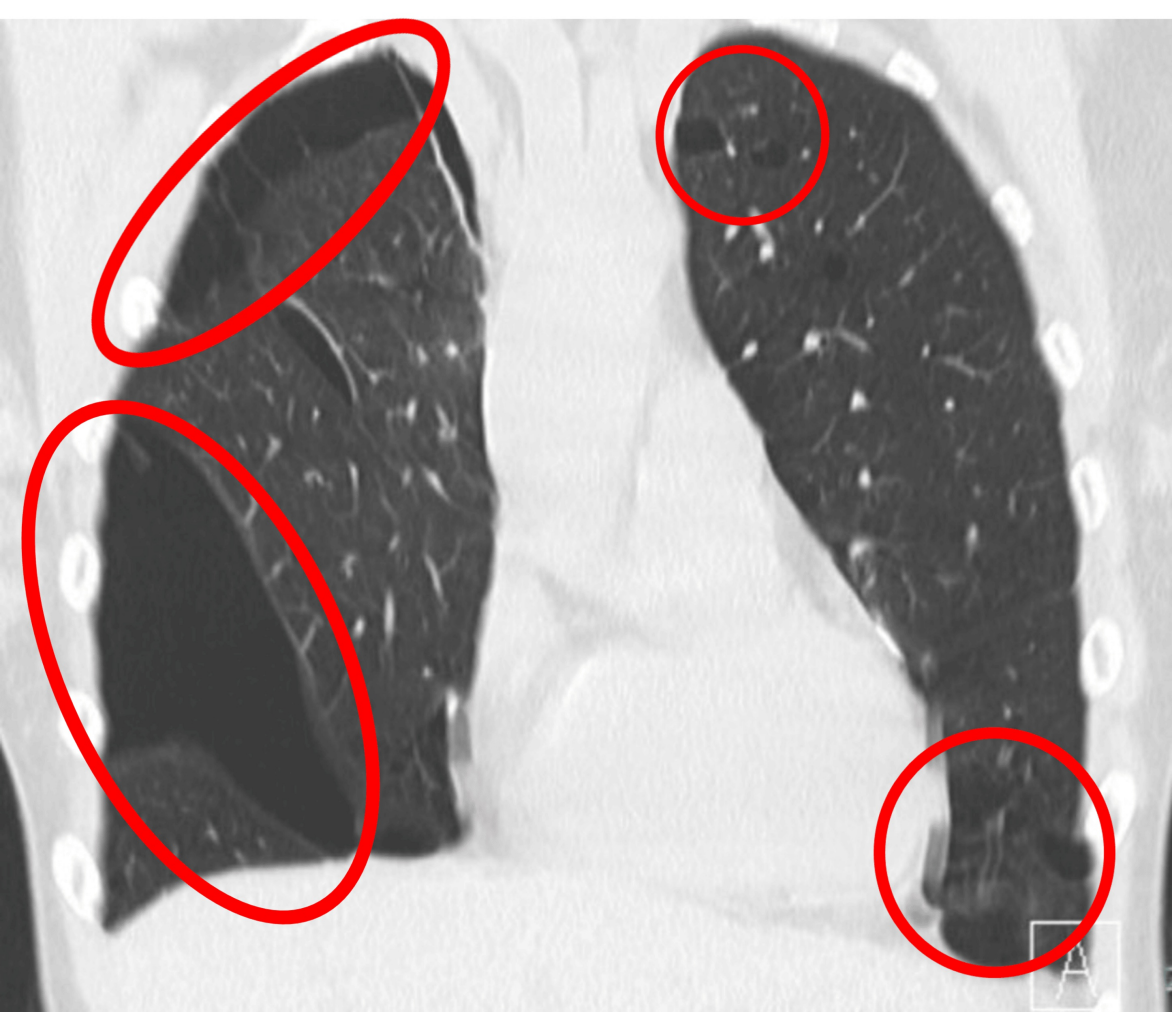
Preoperative chest CT (coronal) showing multiple pulmonary cysts. A chest CT shows numerous bilateral pulmonary cysts (red circles and ovals). CT, Computed Tomography
